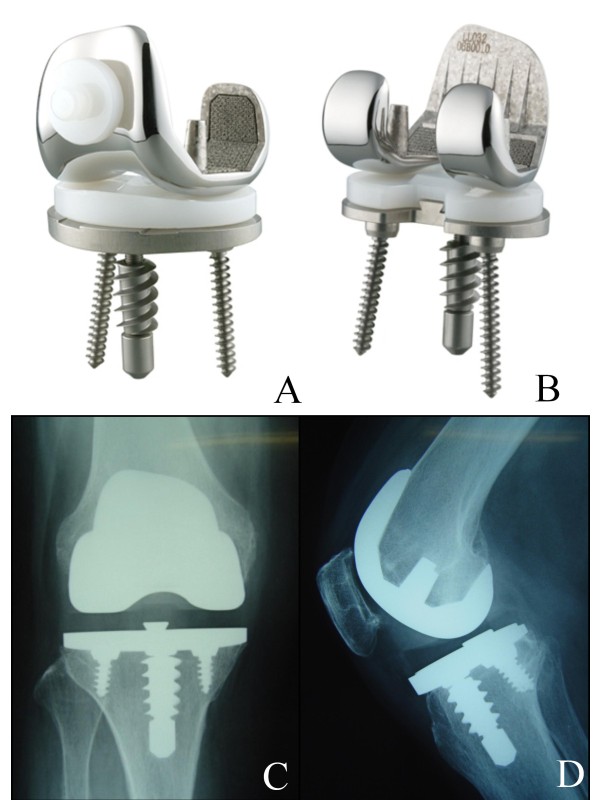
A: Anterior view of the Hi-tech knee II prosthesis CR type component. Note the polyethylene geometry with flat surface. B: Posterior view of the Hi-tech knee II prosthesis CR type component. Femoral component demonstrating the porous titanium coating and have 6 fins at anterior portion. C: Anteroposterior radiograph of Hi-tech knee II prosthesis CR type. D: Lateral radiograph of Hi-tech knee II prosthesis CR type.
